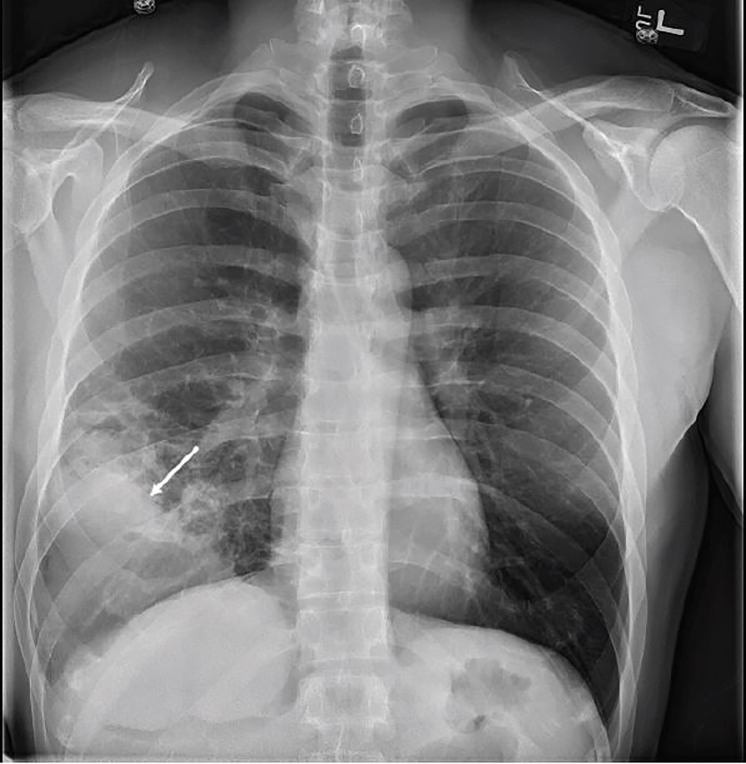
Chest radiograph demonstrating a consolidation (arrow).
